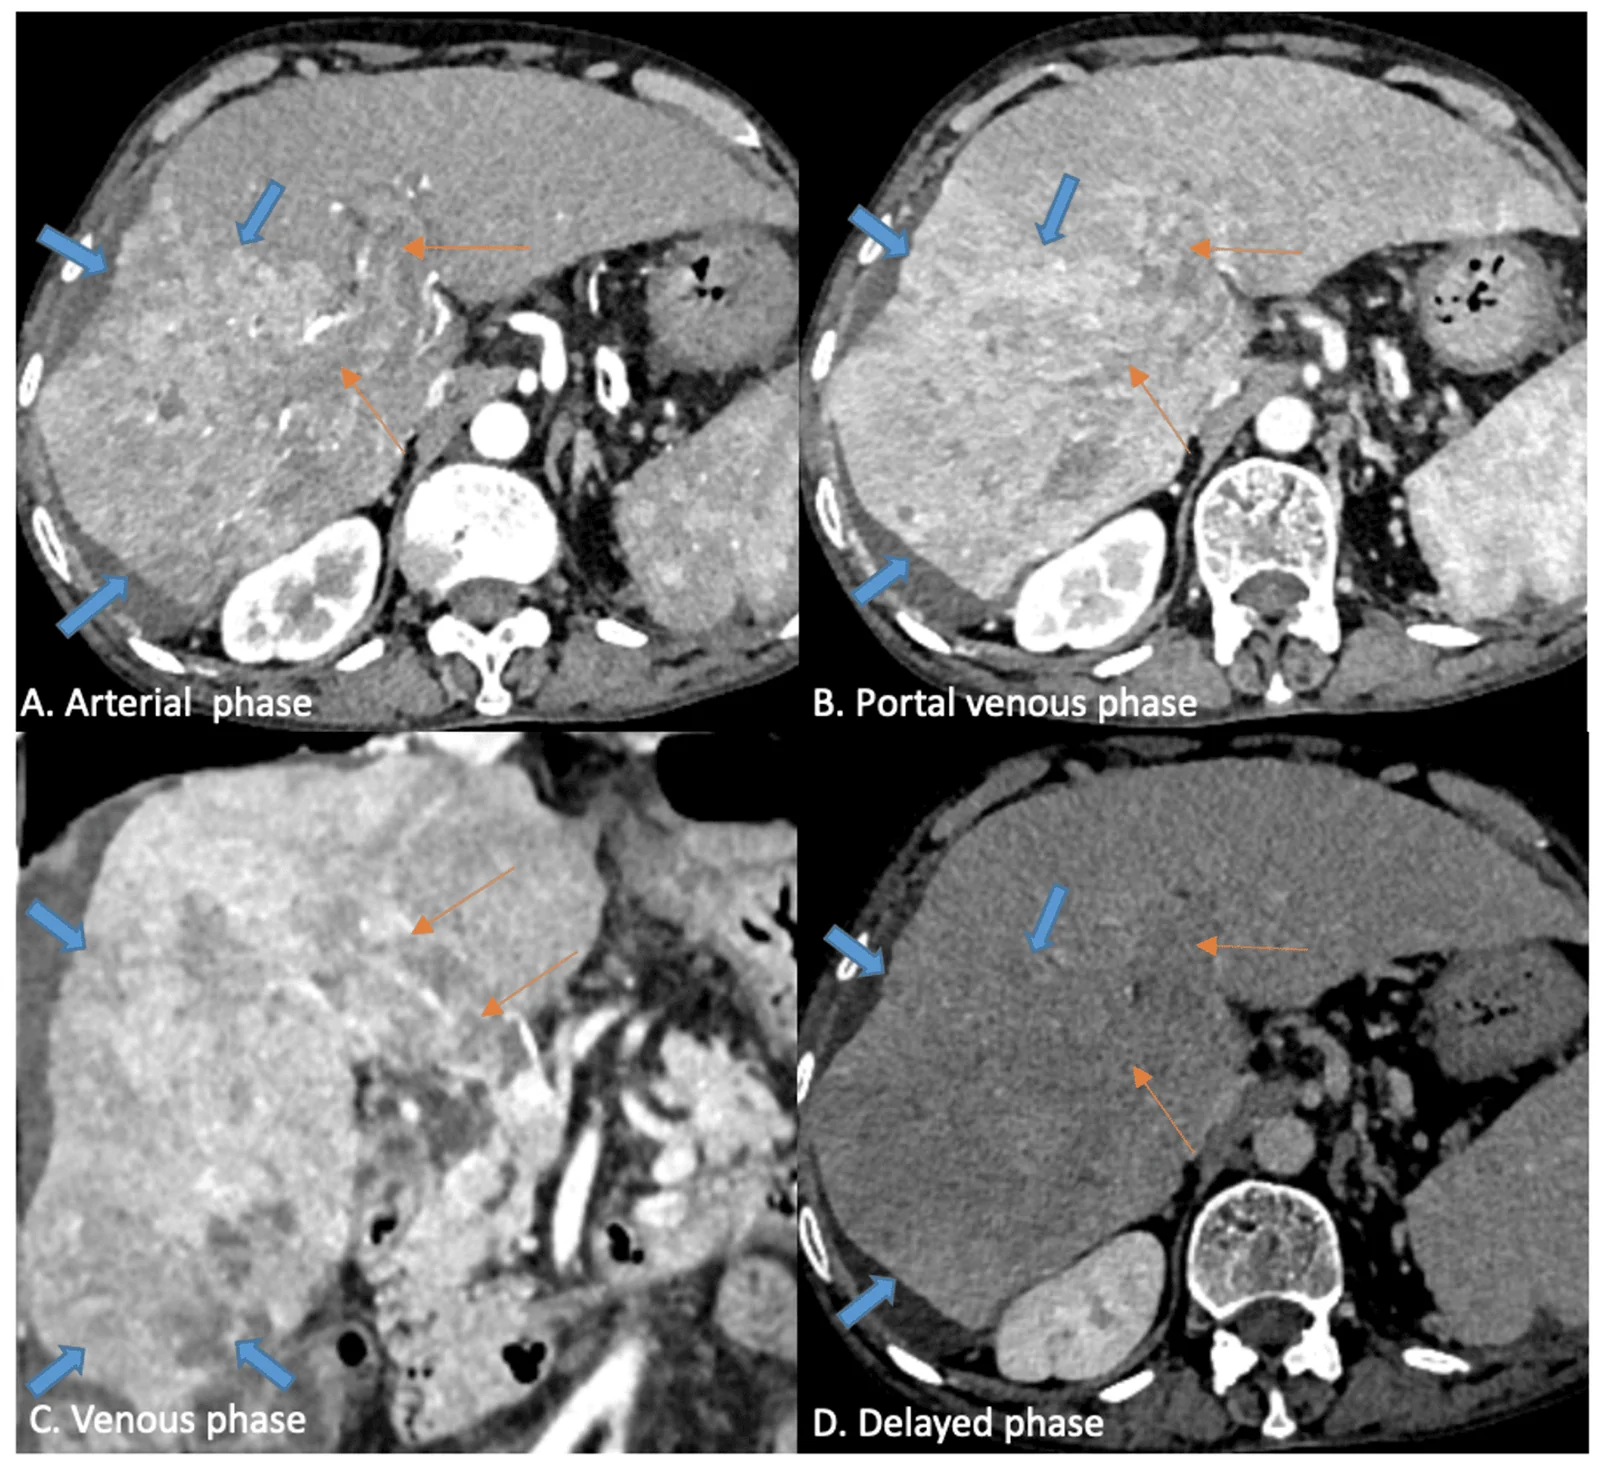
LR-TIV with LR-5 observation on multiphasic contrast-enhanced CT. Multiphasic contrast-enhanced CT images obtained during the arterial (A), portal venous (B), venous (C), and delayed (D) phases, including axial images (A, B, and D) and a coronal reconstruction (C), demonstrate enhancing soft tissue within the main, right, and left portal veins (orange arrows), consistent with LR-TIV. The tumor in the right portal vein is contiguous with a large hepatic observation in the right lobe (blue arrows), demonstrating nonrim arterial phase hyperenhancement, nonperipheral washout on the portal venous (B), venous (C), and delayed (D) phases, and imaging features consistent with an LR-5 observation in a patient with chronic liver disease.
